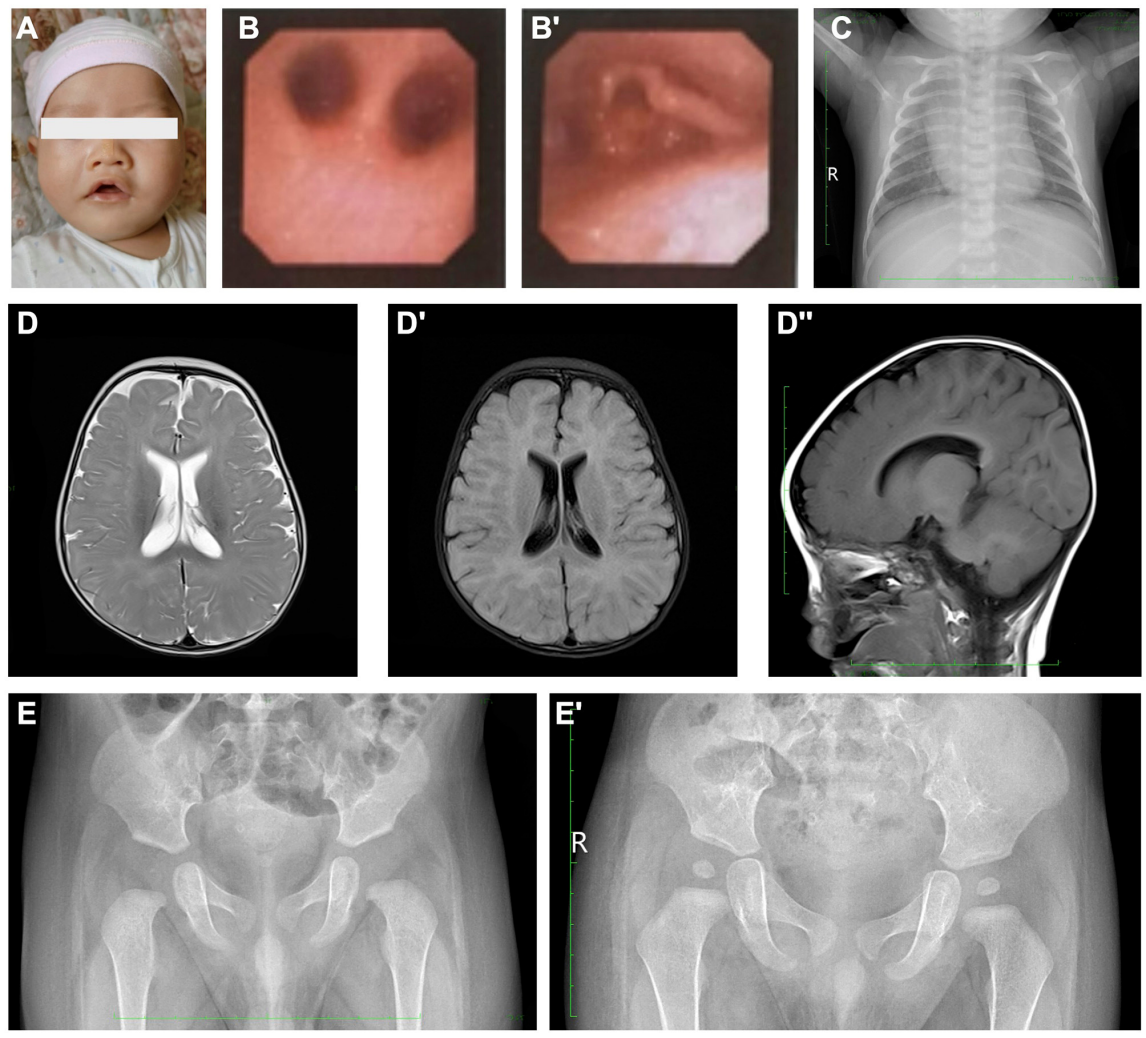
Clinical images presentation of the proband. (A) Abnormal facial appearance of the proband. (B, B') Bronchoscopy examinations indicated a negative finding of pharyngeal and bronchus stenosis, while only laryngomalacia could be observed. (C) Chest X-ray of the proband. (D–D”) Cerebral MRI demonstrated normal morphology and signaling. (E, E') Pelvic X-ray examination. Image (E) was taken at 3-month-old, and (E') was taken at 1.5-year-old.
